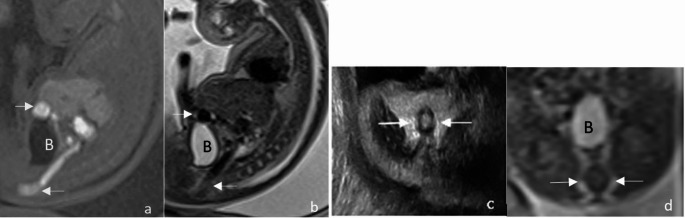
Normal morphology of the distal digestive tract Midline sagittal T1- (a) and T2-W (b) MR images at 30GW show normal meconium filling the colon and rectum (arrows), homogeneously hyperintense on T1- and hypointense on T2-W images. US (c) and axial T2-W MR (d) images of the fetal perineum at 32GW show the normal anal complex (arrows) with central located “target sign”. B: Bladder
